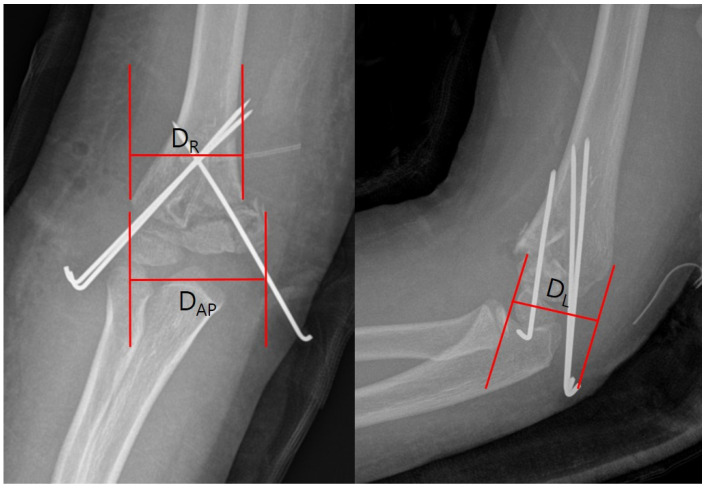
Measurements and equation of rotational angle. The dimensions of the distal fracture line (D_AP_) and the proximal fracture line (D_R_) were measured on AP radiographs. The distal fracture line (D_L_) was also measured on lateral radiographs. The rotational angle was calculated using the following formula: rotation angle = arc cos [(D_R_ − D_L_)/(D_AP_ − D_L_)].
